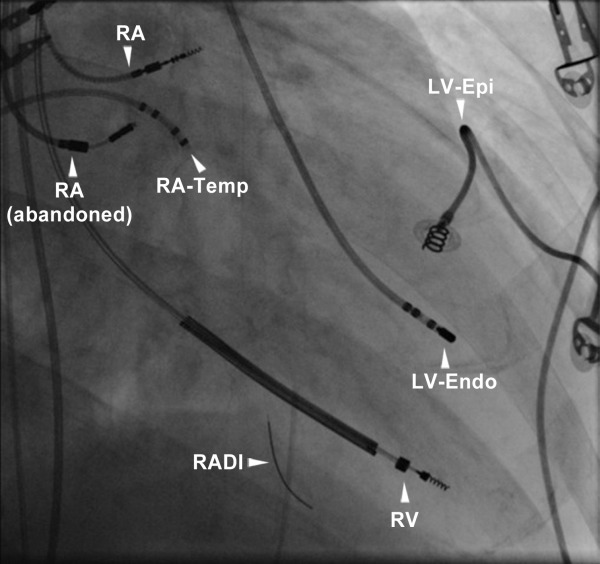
X-ray detail of the invasive hemodynamic pacing study setup. The right atrial (RA), right ventricular (RV) and LV epicardial (LV-Epi) lead of the implanted system are shown, together with the temporary right atrial (RA-Temp) and LV endocardial electrode (LV-Endo). The RADI pressure wire is located in the LV cavity
